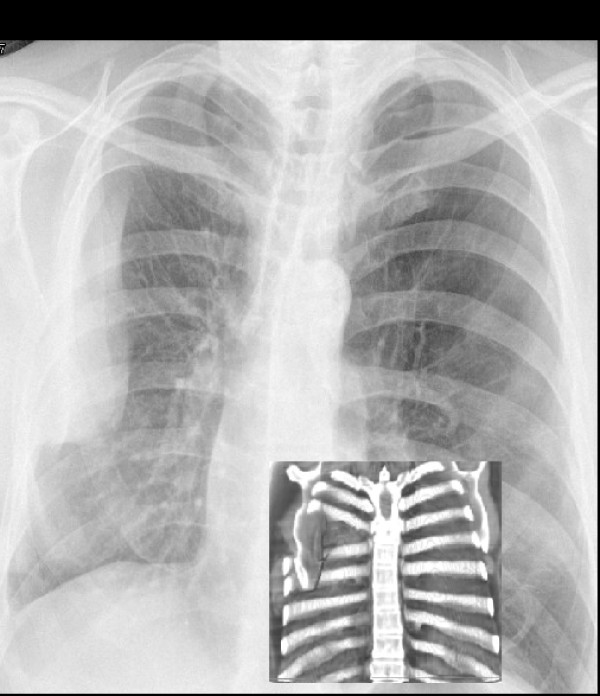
Chest radiograph showing a right peripheral density following dislocation of the scapula.
